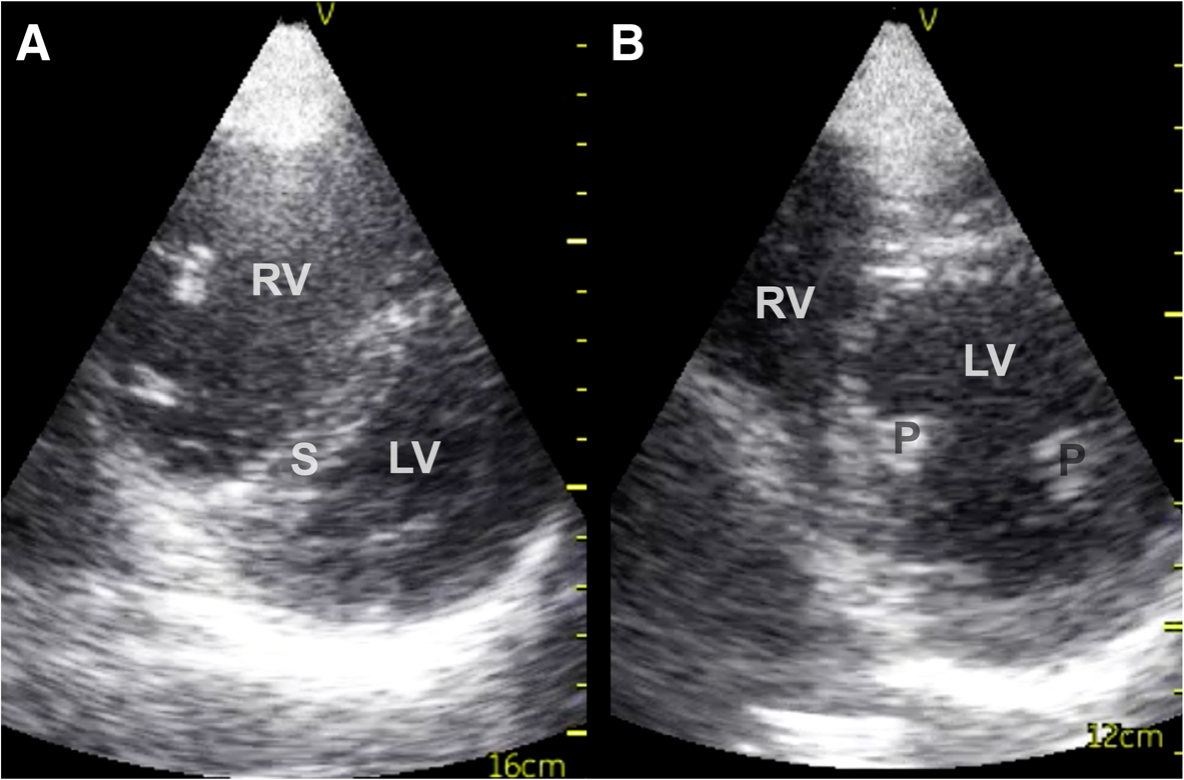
Parasternal short axis view – pulmonary embolism and normal. A: Parasternal short axis view – pulmonary embolism. Notice the bulging of the septum into a typical D-shaped left ventricle. B: Parasternal short axis view - normal. RV = Right Ventricle, LV = Left Ventricle, S = Septum, P = Papillary muscles.
